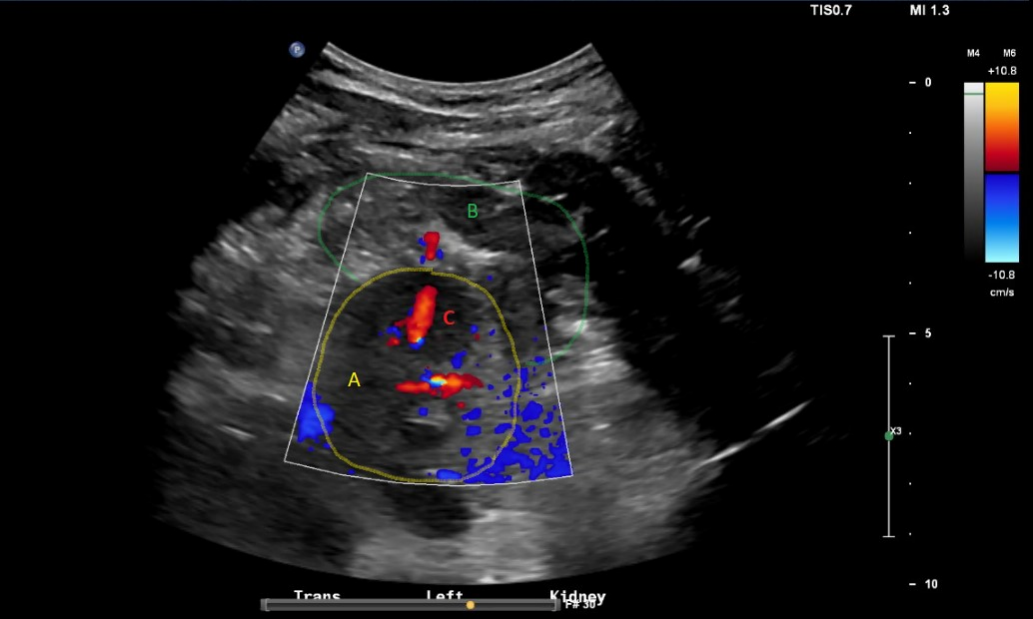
(A) Left kidney, lower pole; (B) hematoma; (C) Doppler flow signal from vascular jet. Ultrasound at 90 minutes after biopsy. Persistent bleeding evidenced by ongoing tract of turbulent flow on color Doppler. Hematoma size 7.9 x 2.3 x 4.9cm.
